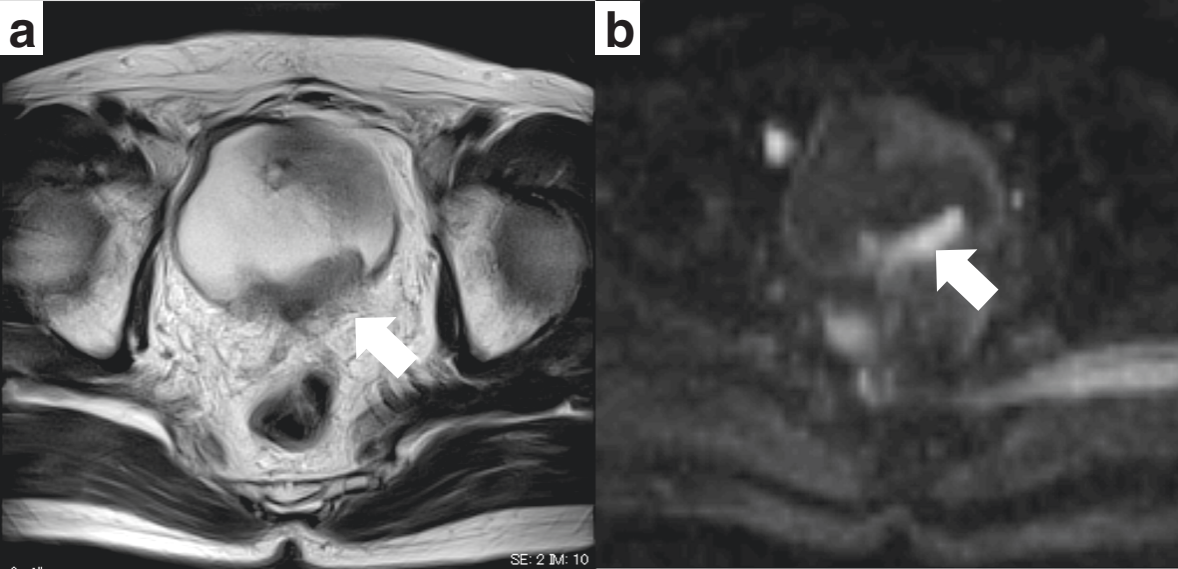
Magnetic resonance imaging findings in (a) T2-weighted and (b) diffusion-weighted images. The mass was believed to be invasive outside of the bladder wall (*arrow*)
